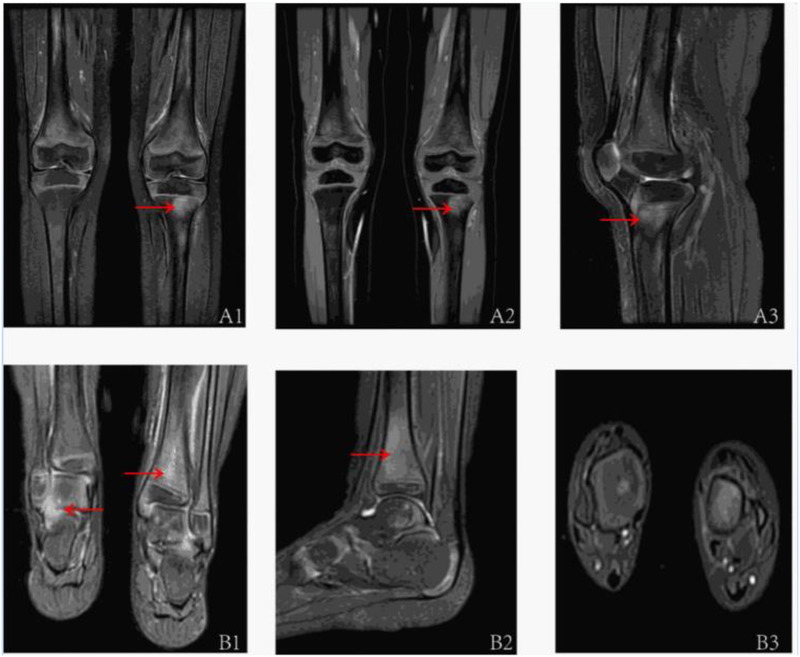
The MRI assessments of the children's joints are as follows: A1–A3 pertain to the MRI evaluations of both knee joints (A1: anterior; A2: anterior; A3: left lateral). The T2IDEAL WATER images revealed bone marrow edema in both distal femurs and the proximal left tibia. B1–B3 correspond to the MRI evaluations of both ankle joints of the patient (B1: Posterior; B2: Left Lateral; B3: Sagittal Plane), demonstrating abnormal bone signals at the distal tibia and swelling of the surrounding soft tissues, as observed in the T2IDEAL WATER images.
